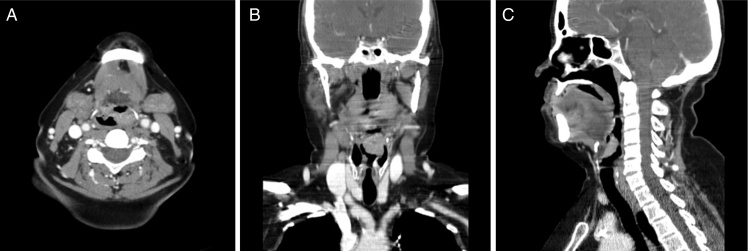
Computed tomography depicting a vegetative lesion in the epiglottis and affecting the left aryepiglottic fold in the axial (A), coronal (B), and sagittal (C) views.
